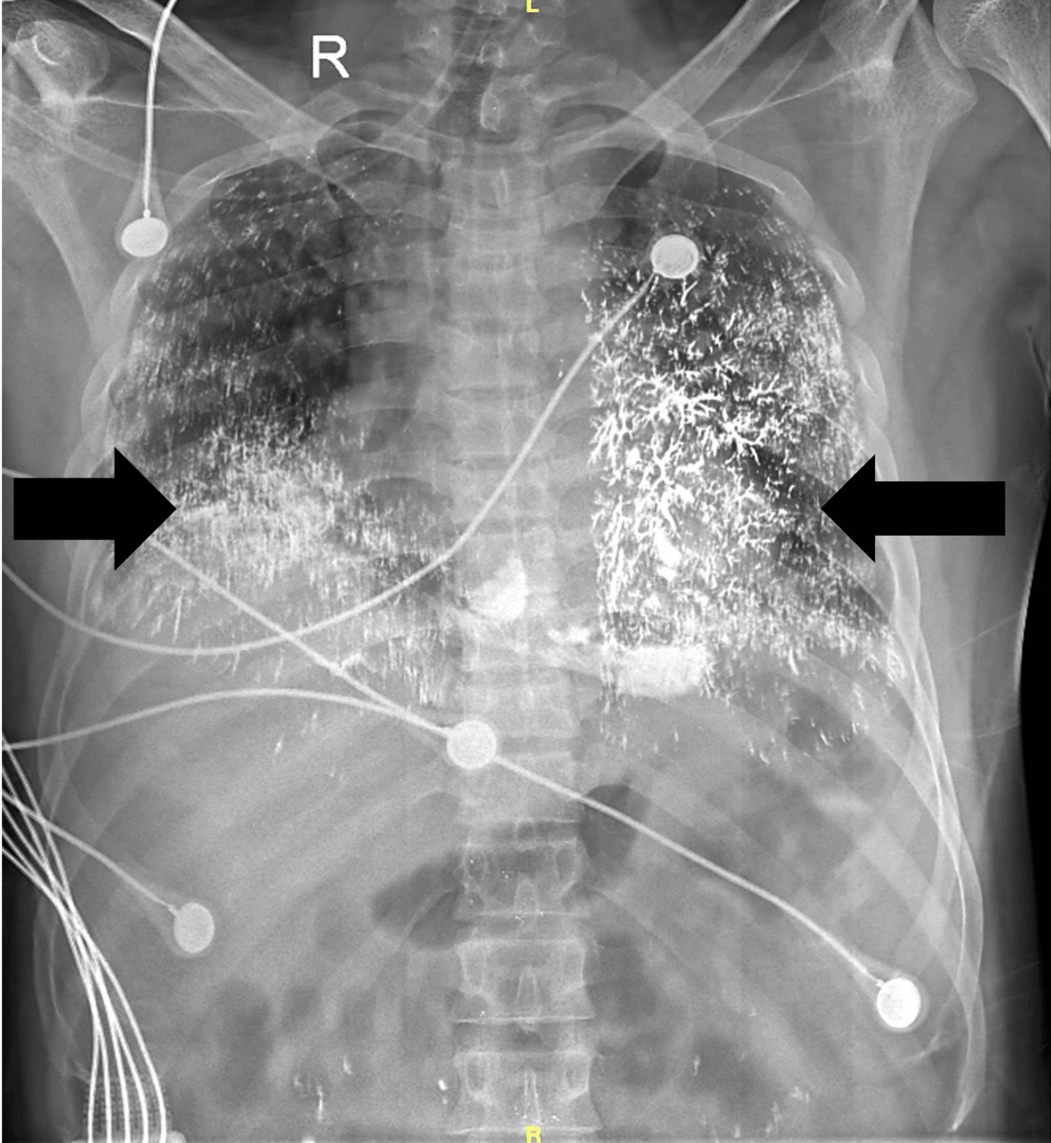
Chest radiograph shows multiple foci of rounded opacities throughout bilateral lung fields in a branching pattern (black arrows) with a history of elemental mercury poisoning.
